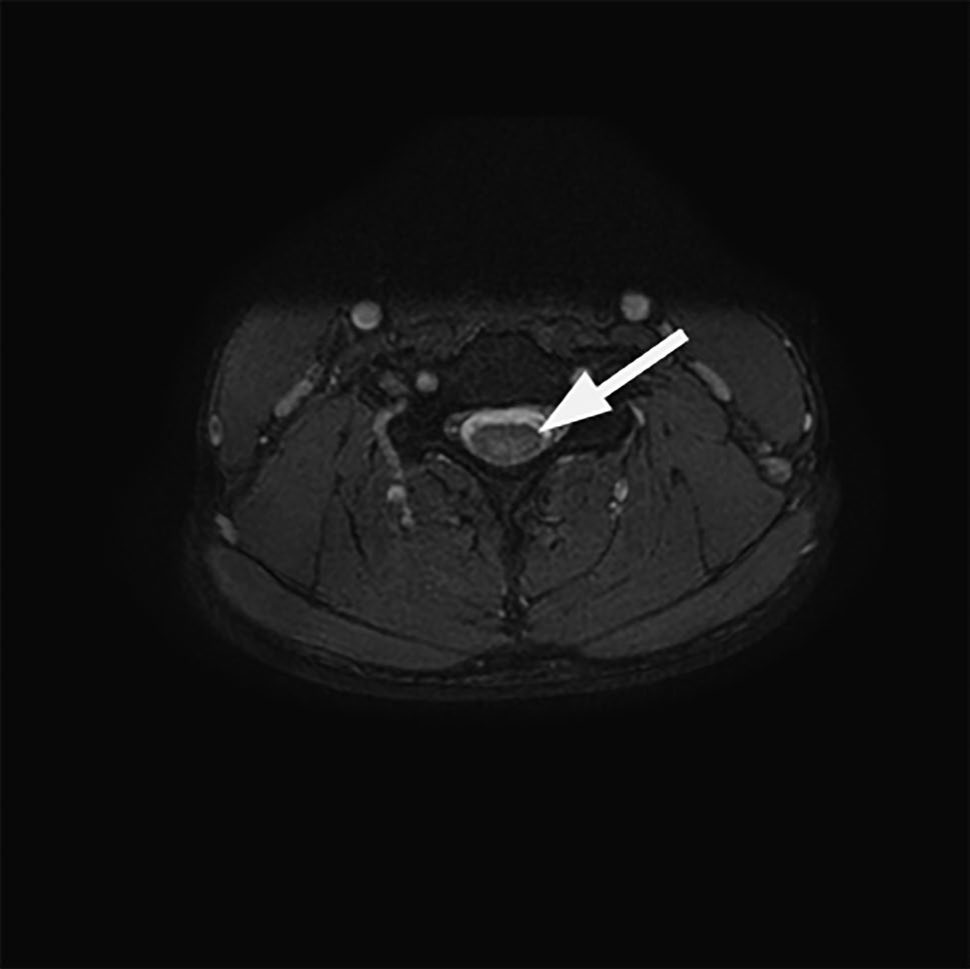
T2-weighted MRI; axial views showing increased signal in the dorsal columns at C5 (white arrow)
